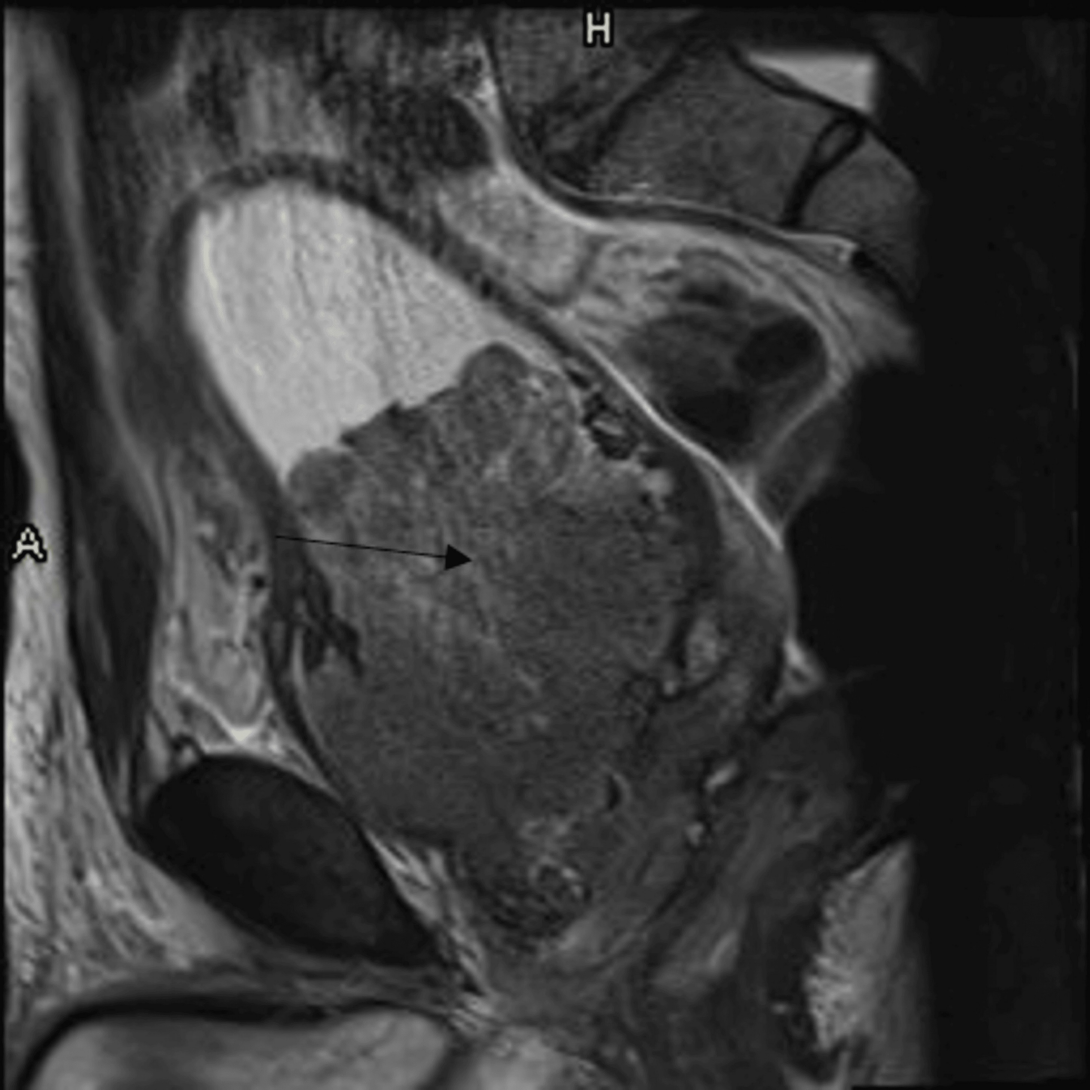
Prostate MRI The previously seen multiple non-enhancing cystic lesions in the pelvis have completely resolved in the interval. MRI: magnetic resonance imaging
